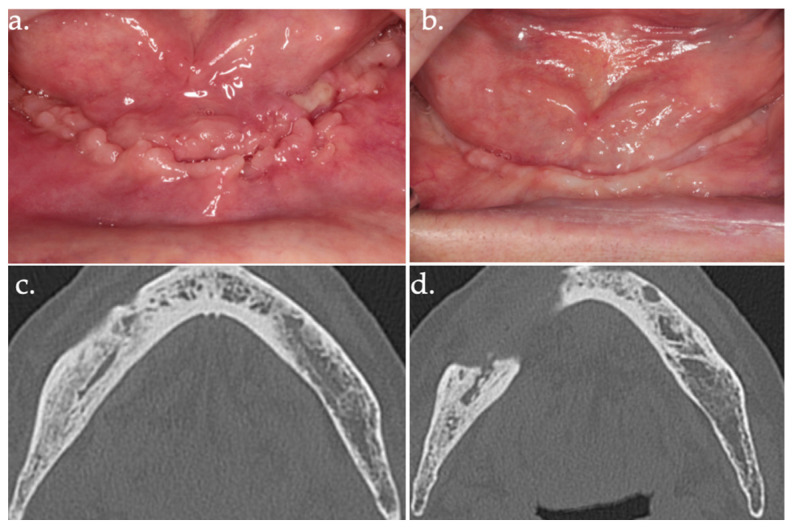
24 days follow-up (a); 17 months follow-up (b); follow-up CT scan at 18 months after surgery (c,d).
